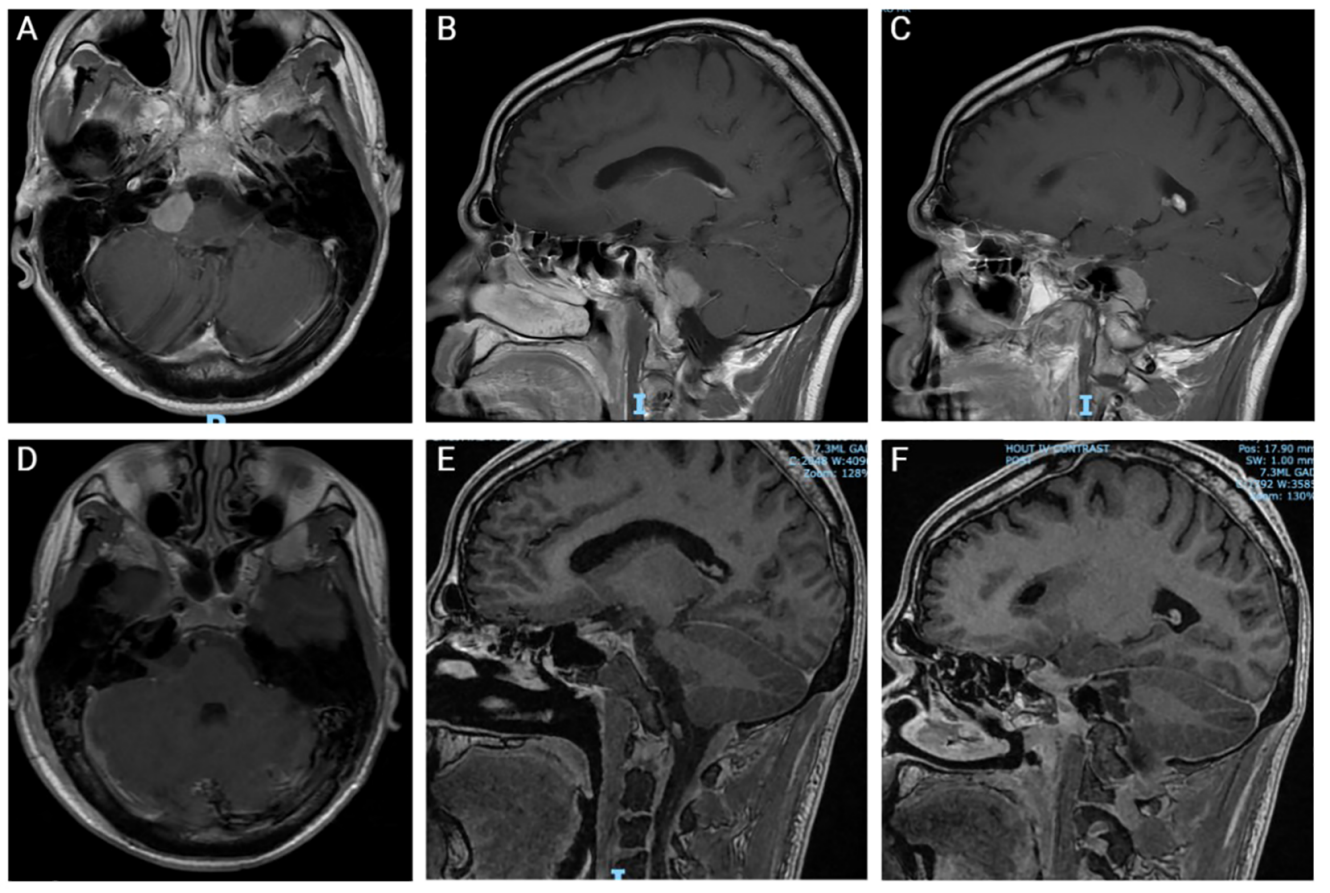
Pre- and post-operative Magnetic Resonance Imaging (MRI). (A-C) Preoperative coronal (A) and sagittal (B, C) MRI with contrast. (D-F) Postoperative coronal (D) and sagittal (E, F) MRI without contrast.
